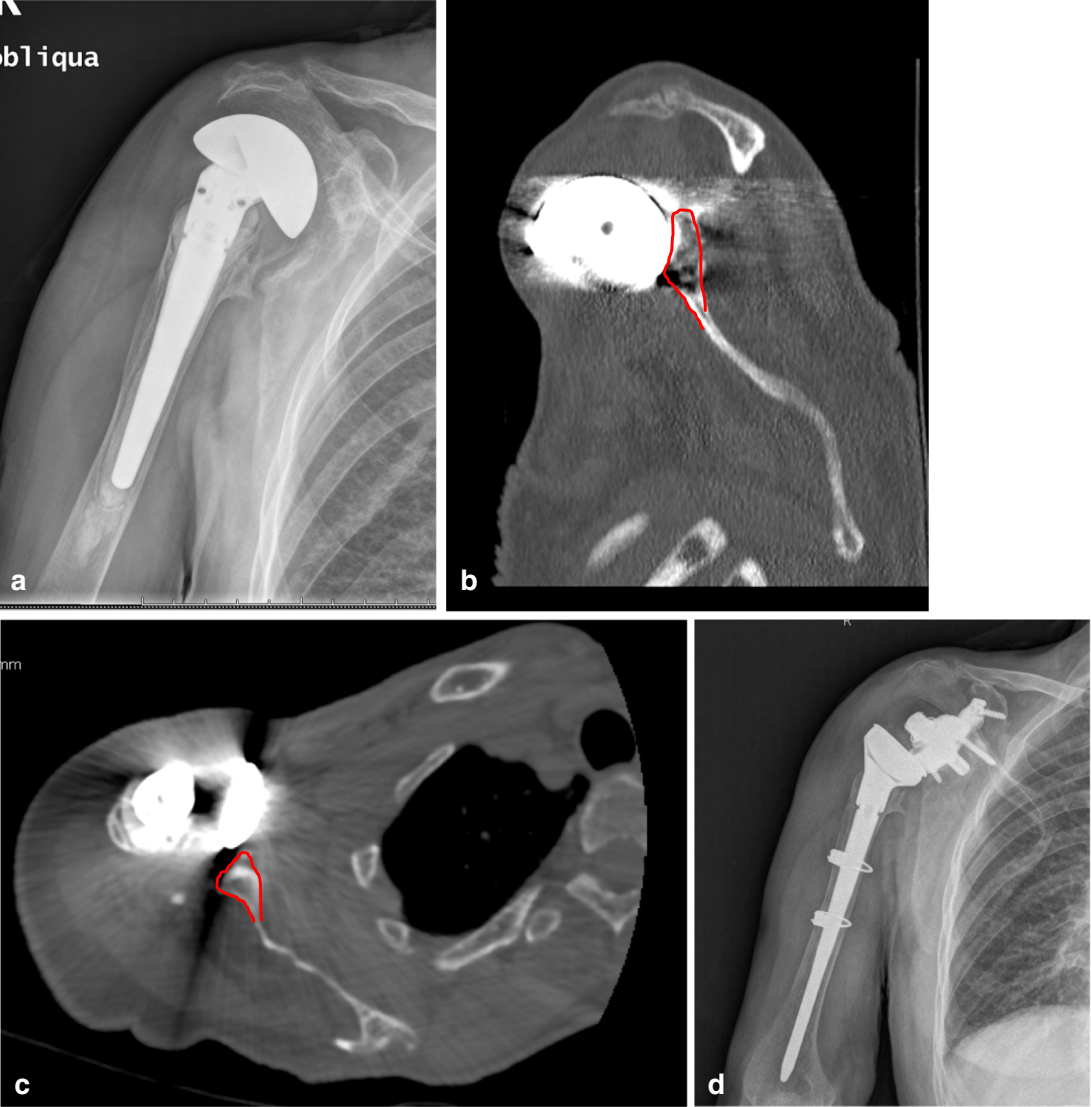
a Preoperative X-rays, b preoperative sagittal plane CT scan, c preoperative oblique plane CT scan, d postoperative X-rays
